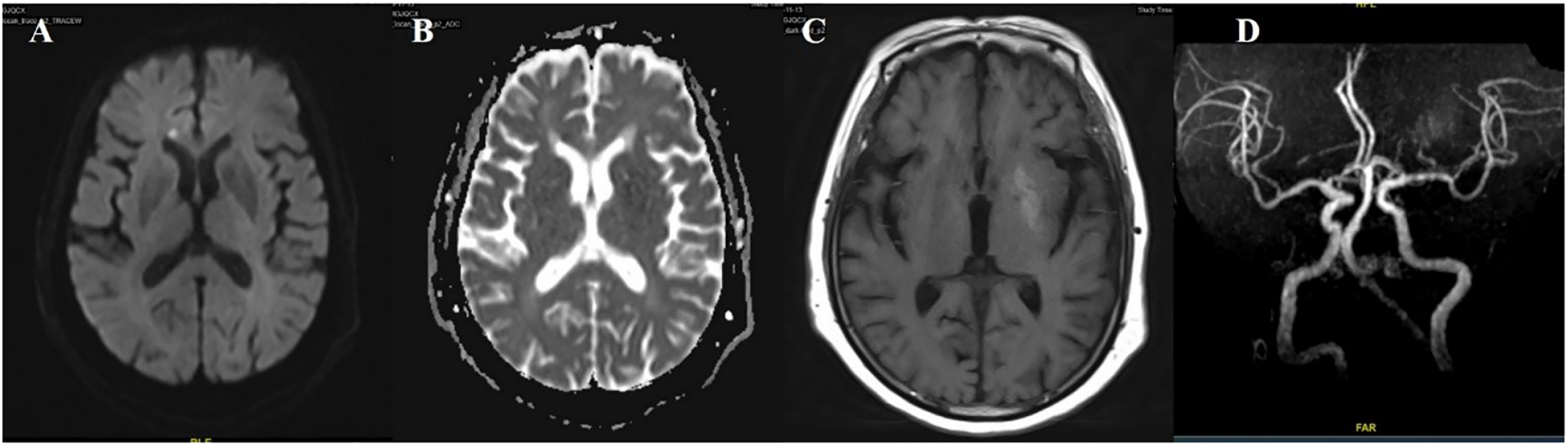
Case 5. DWI sequence shows high signals in corpus callosum (A). The corresponding area (B) show low signals on the ADC map. (C) Brain MR T1 image demonstrated hyperintensity in the left putamen. (D) Brain MRA indicated mild-moderate stenosis of left MCA. .
